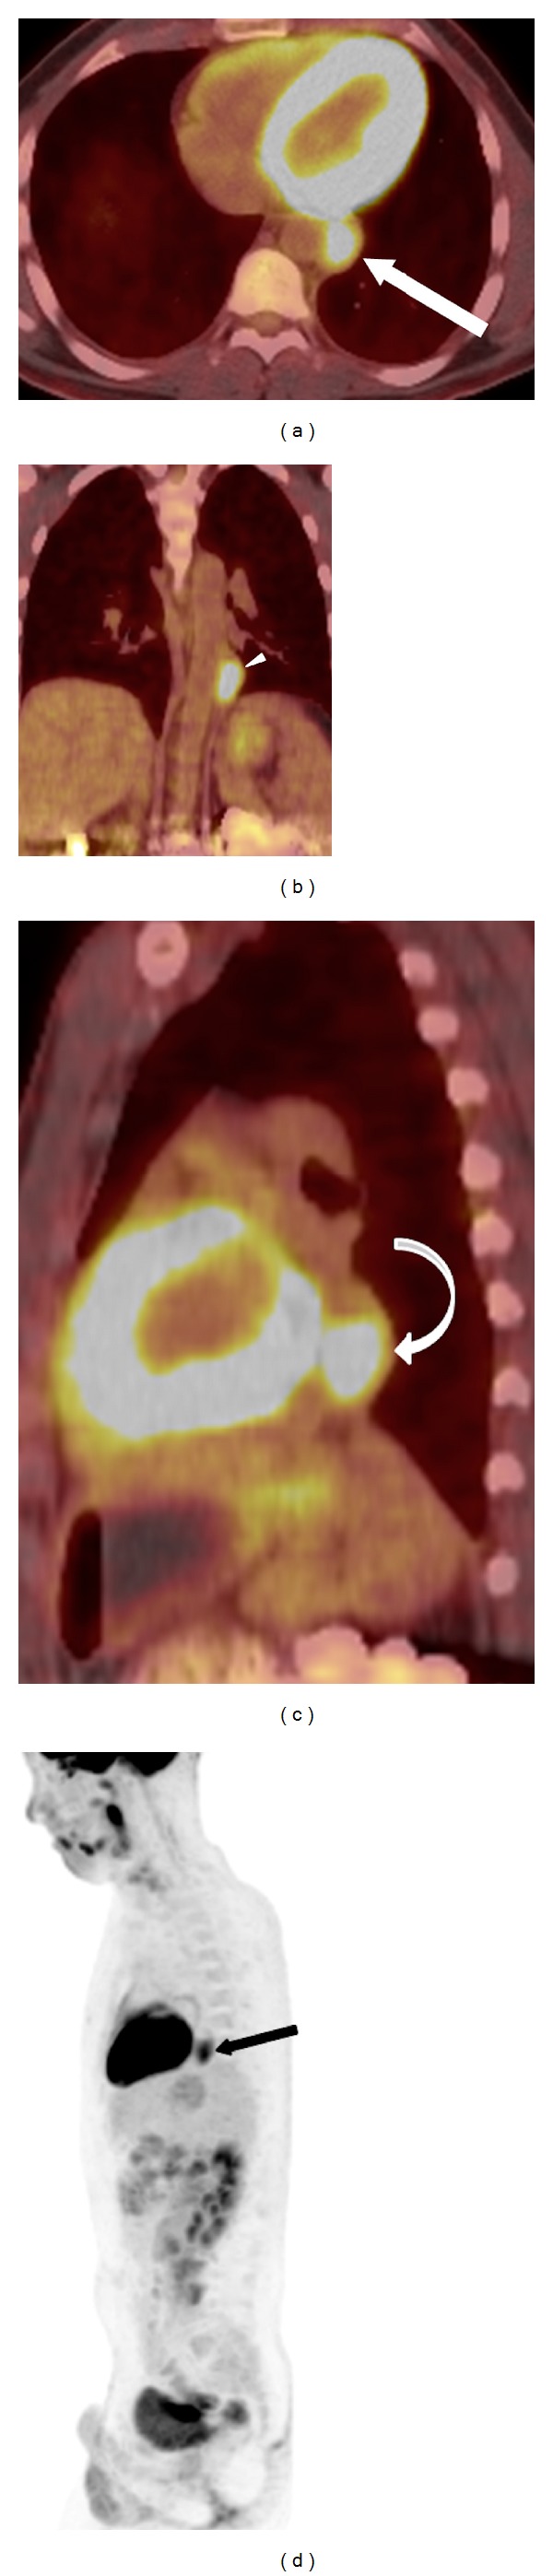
(a) PET utilizing fluorine-18 fluorodeoxyglucose (F-18 FDG) axial fused CT image showing the increased FDG avidity associated with the paraesophageal mass (arrow). Images obtained on a Siemens Biograph PET CT 64 slice after administration of 12.31 mCi F-18 FDG. Total DLP 447 mGycm. (b) Coronal multiplanar reformatted fused PET CT image showing the FDG avid mass adjacent to the thoracic aorta (arrowhead). (c) Sagittal multiplanar reformatted fused PET CT image showing the FDG avid retrocardiac mass (curved arrow). (d) Maximal intensity projection (MIP) fused PET CT image showing the retrocardiac mass (black arrow).
